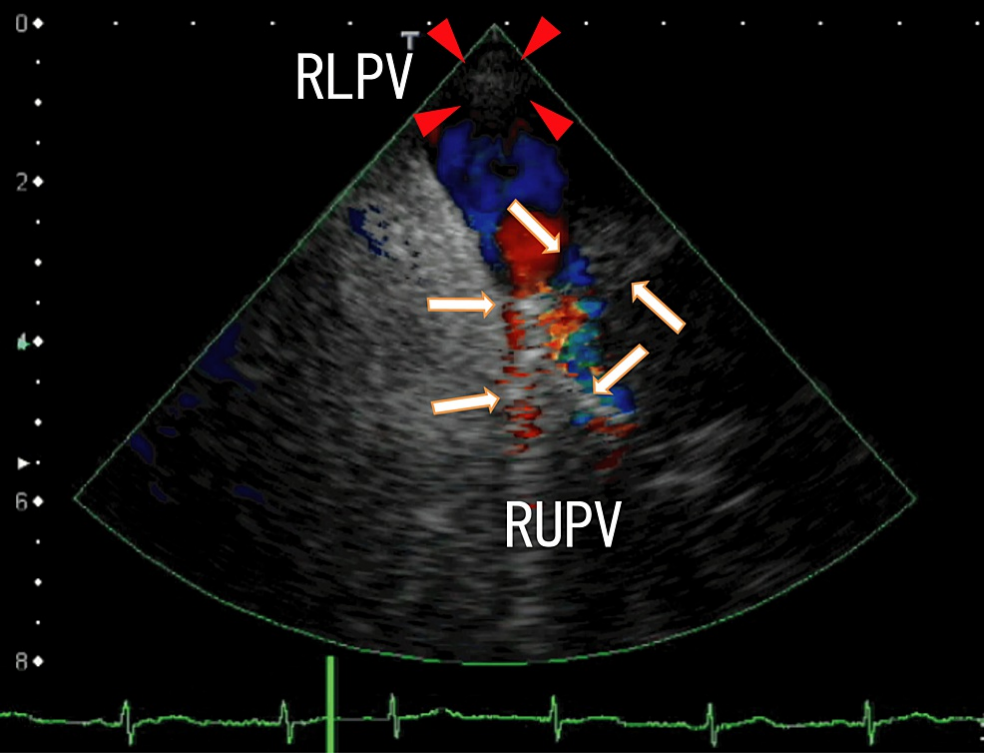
TEE images showing the RUPV thrombi. The arrows indicate the RUPV thrombi, which had two branches. One branch was directed to the left, and the other branch was directed to the right. The LA side of the RUPV thrombi was attached to the left side of the surrounding wall of the exit of the RUPV. In the RUPV, there was a mixture of red, blue, and yellow colors, indicating that there were disturbances in blood flow. The arrowheads indicate the LA thrombi situated around the exit of the RLPV. RLPV, right lower pulmonary vein; RUPV, right upper pulmonary vein; LA, left atrium; TEE, transesophageal echocardiography
